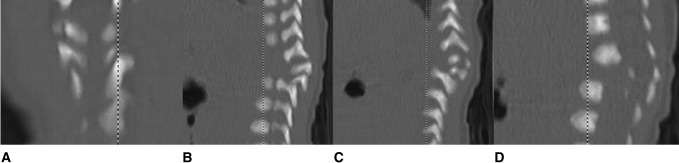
Various CT cuts demonstrating the bilaterial posterior hemivertebrae (A-D). (Reproduced with permission from the Children’s Orthopaedic Center, Los Angeles, CA)
